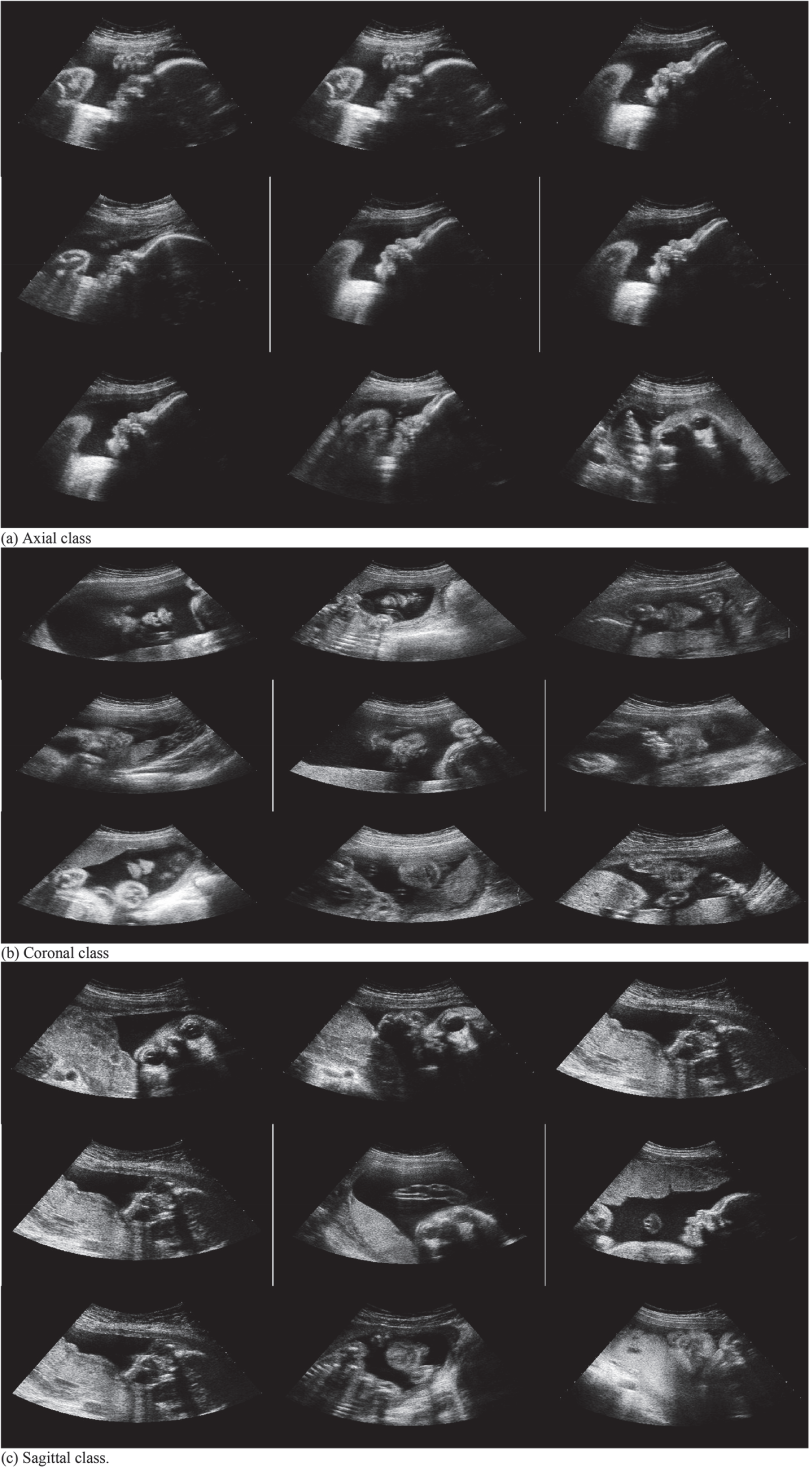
Top similar ultrasound images of the input sample images of (a) axial class (b) coronal class (c) sagittal class. The first one is the input query images and the remaining images are retrieved based on the similarity with the query image.
